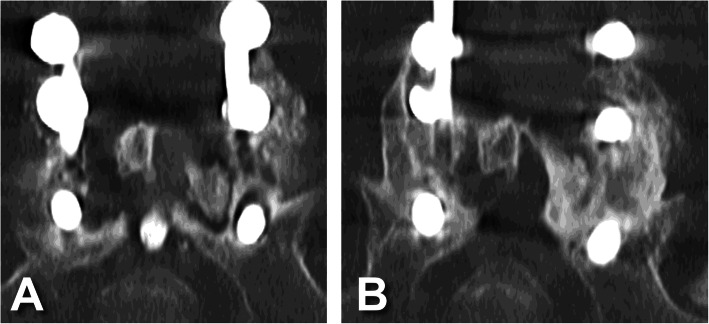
Representative CT scans of a female patient who is a smoker in her 30s. A Preoperative coronal view showing loose S1 pedicle screws with halo around the screw track and no posterolateral fusion mass (Lenke D, definitely not solid with thin fusion masses bilaterally with obvious pseudarthrosis). B Two years postoperative showing posterolateral fusion mass (Lenke A, bilateral stout fusion masses present)
